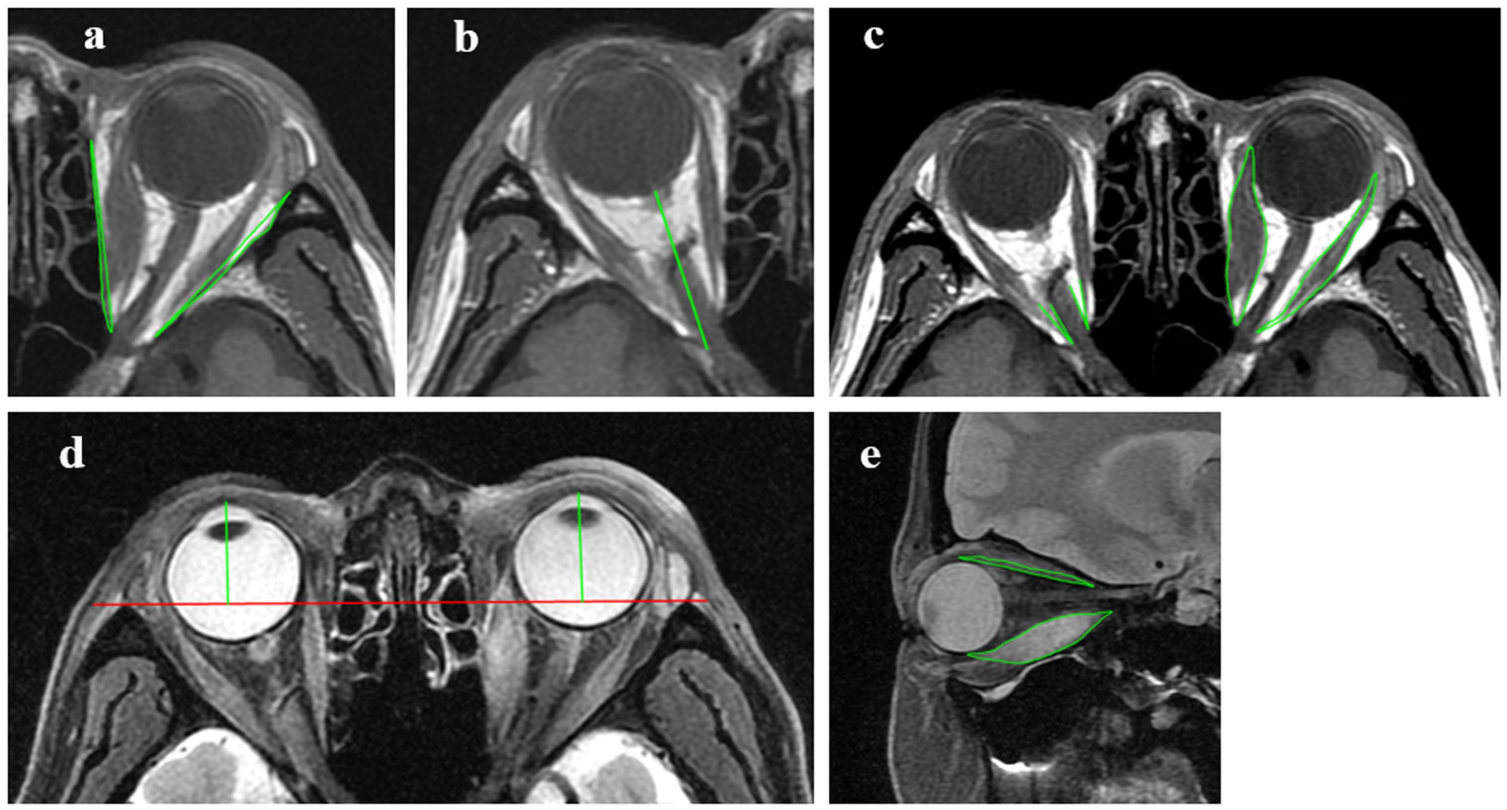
Measurements of MRI indicators. (A) Axial T1WI, measurements of the volume of the medial orbital wall and the volume of the lateral orbital wall. (B) Axial T1WI, measurements of the intraorbital optic nerve stretching length. (C) Axial T1WI, measurements of the medial and lateral rectus area, the angle between the medial rectus and optic nerve and the angle between the lateral rectus and optic nerve. (D) Axial T2W2 FS, measurements of proptosis. (E) Sagittal T2WI FS, measurements of the superior and inferior rectus area.
